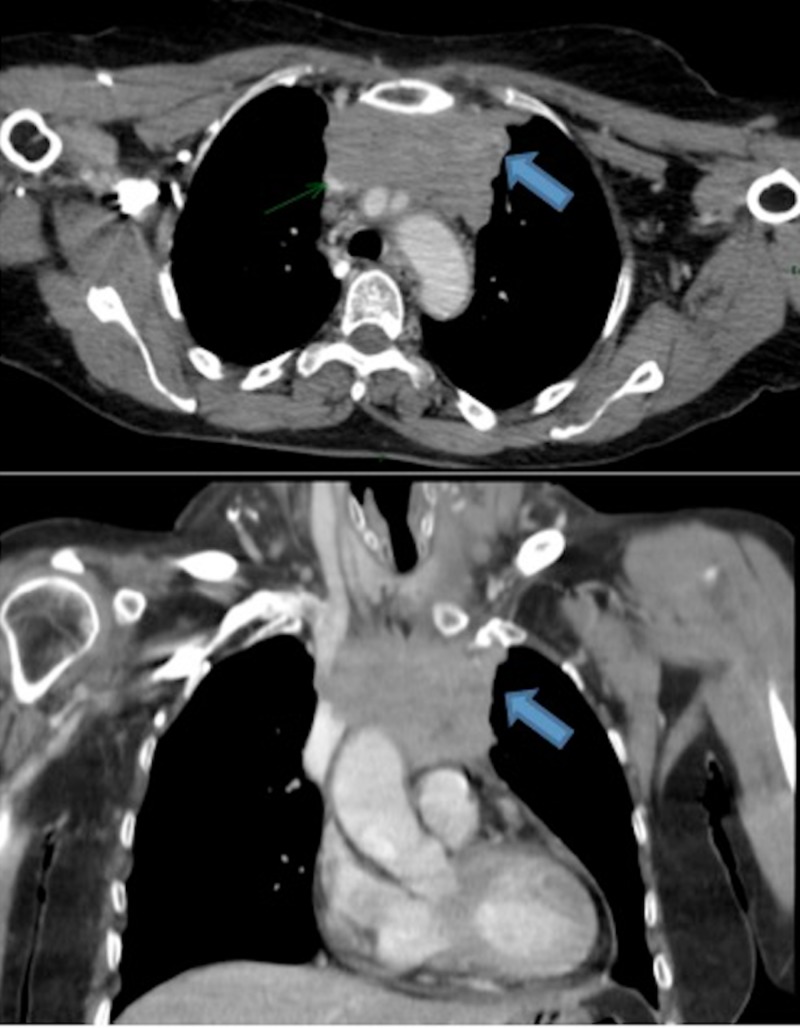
Abdominal-thoracic-cervical CT scan of April 2014. Large, predominantly homogenous mass (discrete left necrotic areas) in the anterior mediastinum with a certain peripheral nodulation that invades the superior vena cava. CT: computed tomography
